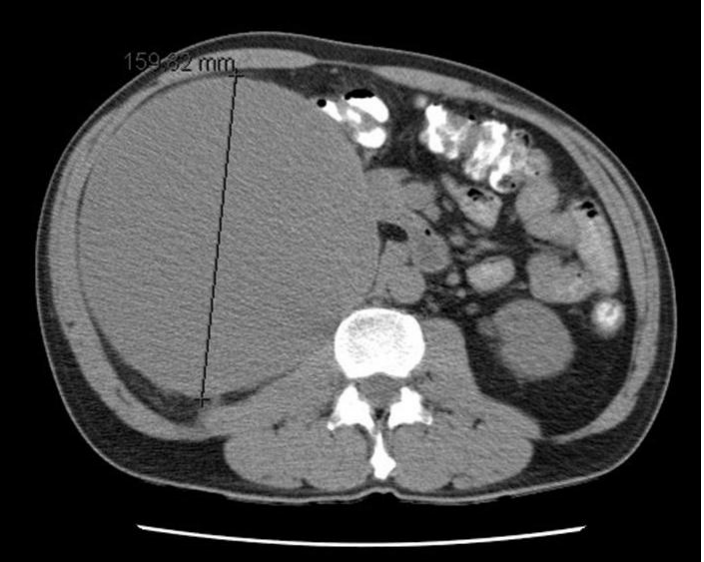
Computed tomography of the abdomen showing large mass lesion.
